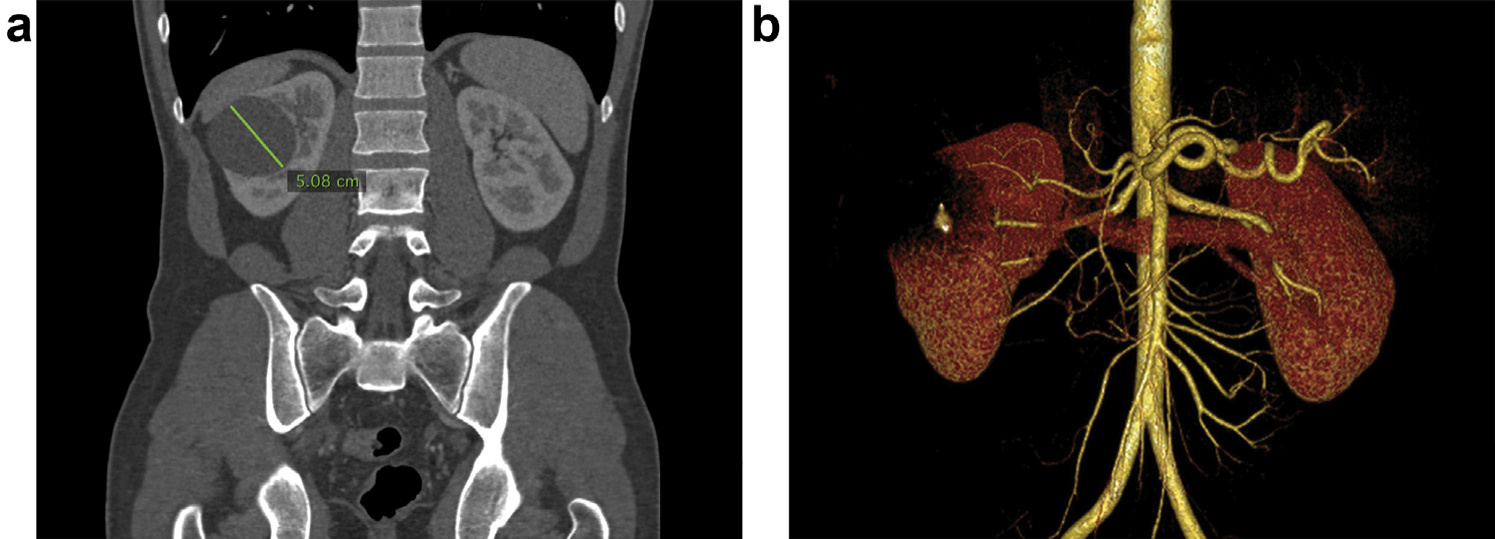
Computed tomography images of the donor, showing (a) a 5.08-cm renal cyst, with (b) an imperceptible wall and benign appearance, classified as Bosniak grade 1.
